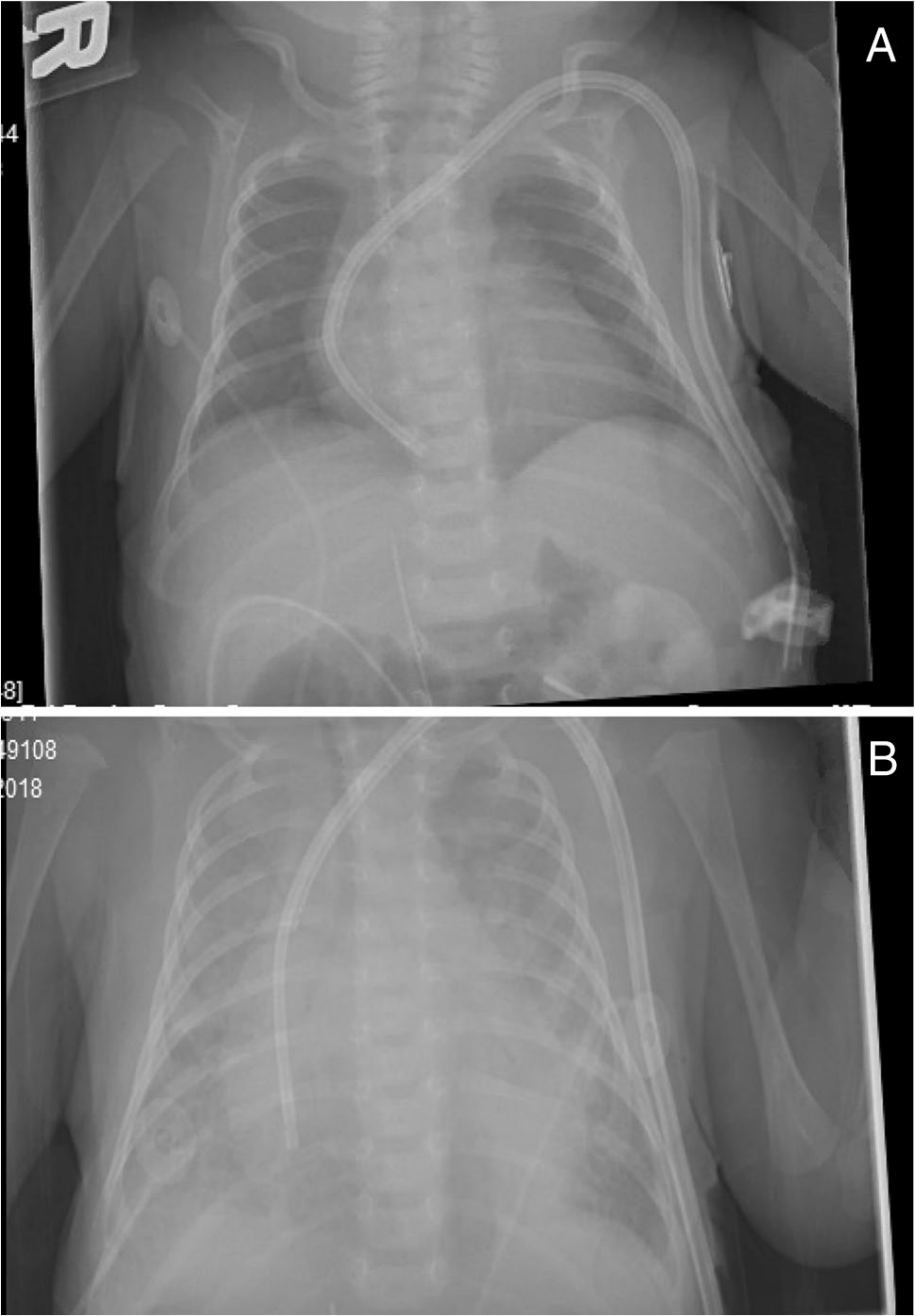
A, B Chest x-ray of infant born with bilateral renal agenesis on day of life 8 (A). Chest x-ray of infant at 3 weeks of age in respiratory distress (B)
